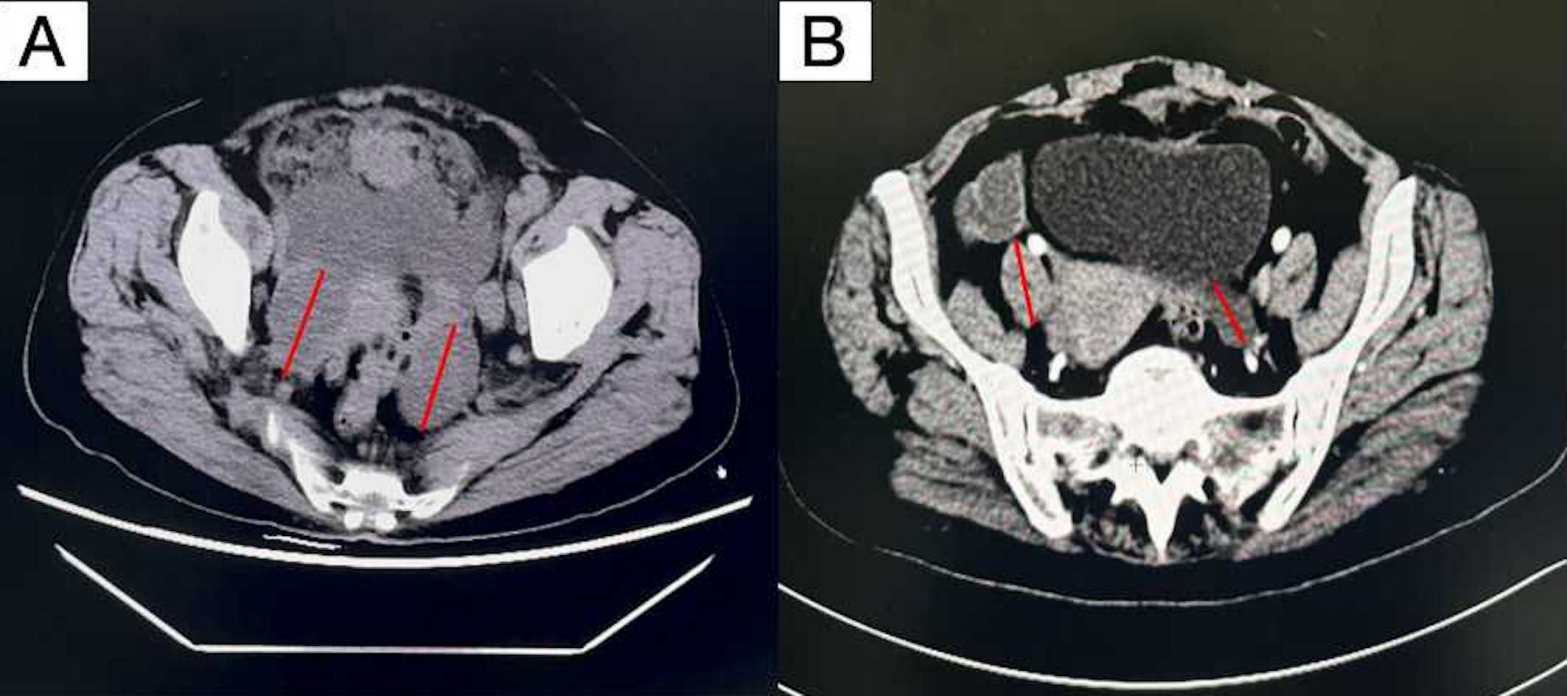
Computed tomography scan showing irregular masses in the bilateral pelvic adnexa area. (A) Before neoadjuvant therapy (B) after neoadjuvant therapy. By comparing (A, B), it can be seen that the tumor bed significantly shrinks after neoadjuvant chemotherapy.
